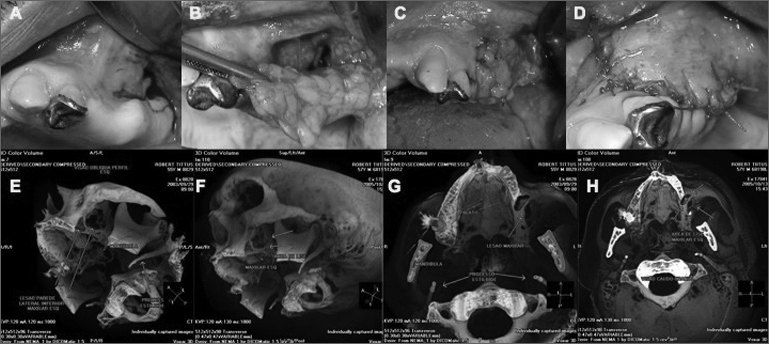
A) Incision surrounding the fistula and two horizontal relief incisions (one anterior and one posterior to the fistula). B) Exposure of the buccal adipose body, which is extended to the bone defect with no tension. C) Suturing the buccal adipose body to the rims of the palatine and vestibular mucosae. D) Suturing the mucosa over the adipose body. E) Preoperative lateral image (3D CT) of the bone defect. F) Postoperative lateral image (3D CT) of the bone defect after one year. G) Preoperative occlusal image (3D CT) of the bone defect. H) Postoperative occlusal image (3D CT) of the bone defect.
